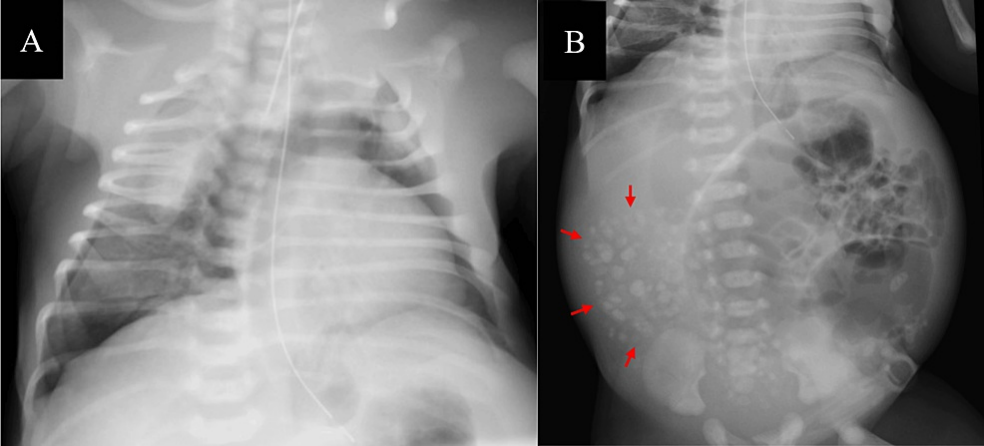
Initial radiographs of the patient (A) Chest X-ray image showing bilateral tension pneumothorax. (B) Abdominal X-ray image showing an enlarged intestinal tract and numerous calcifications in the lower abdomen (arrows).
